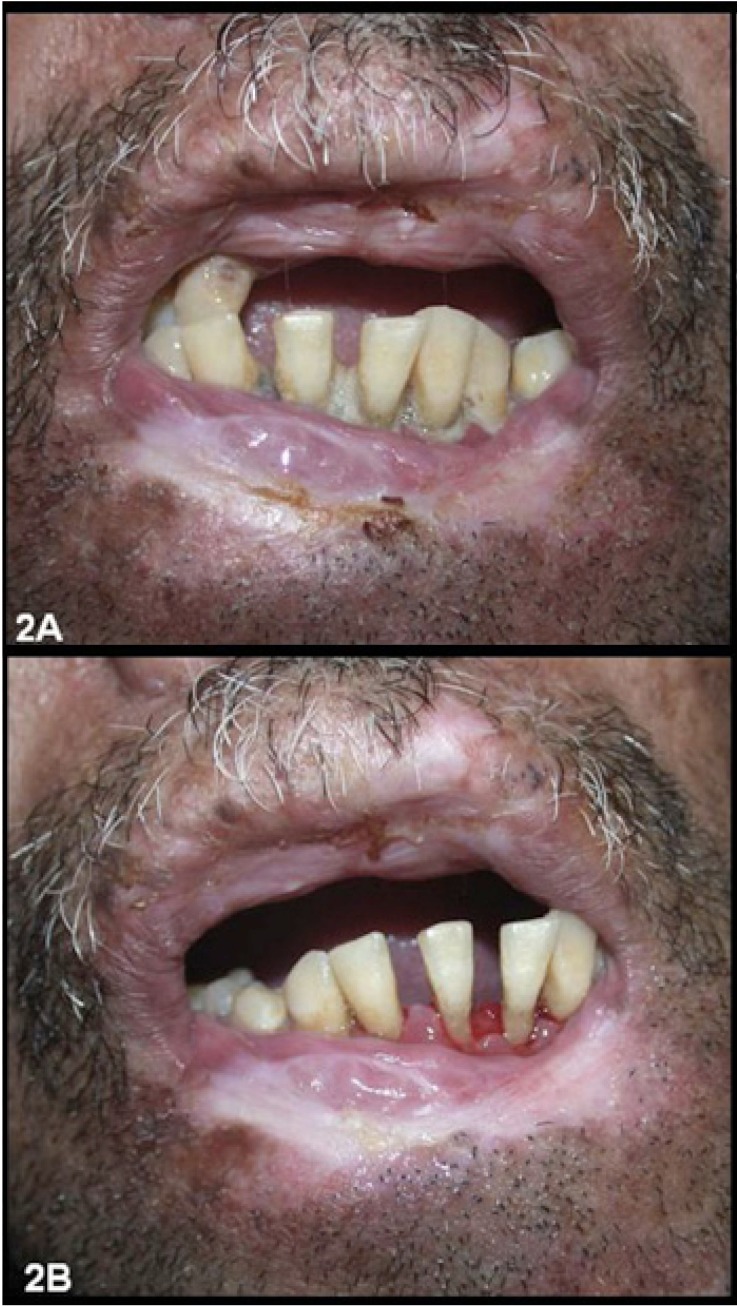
2A. Perioral scars, dental plaque due to poor oral hygiene and microstomy. 2B. Oral condition after dental plaque removal using ultrasound.
